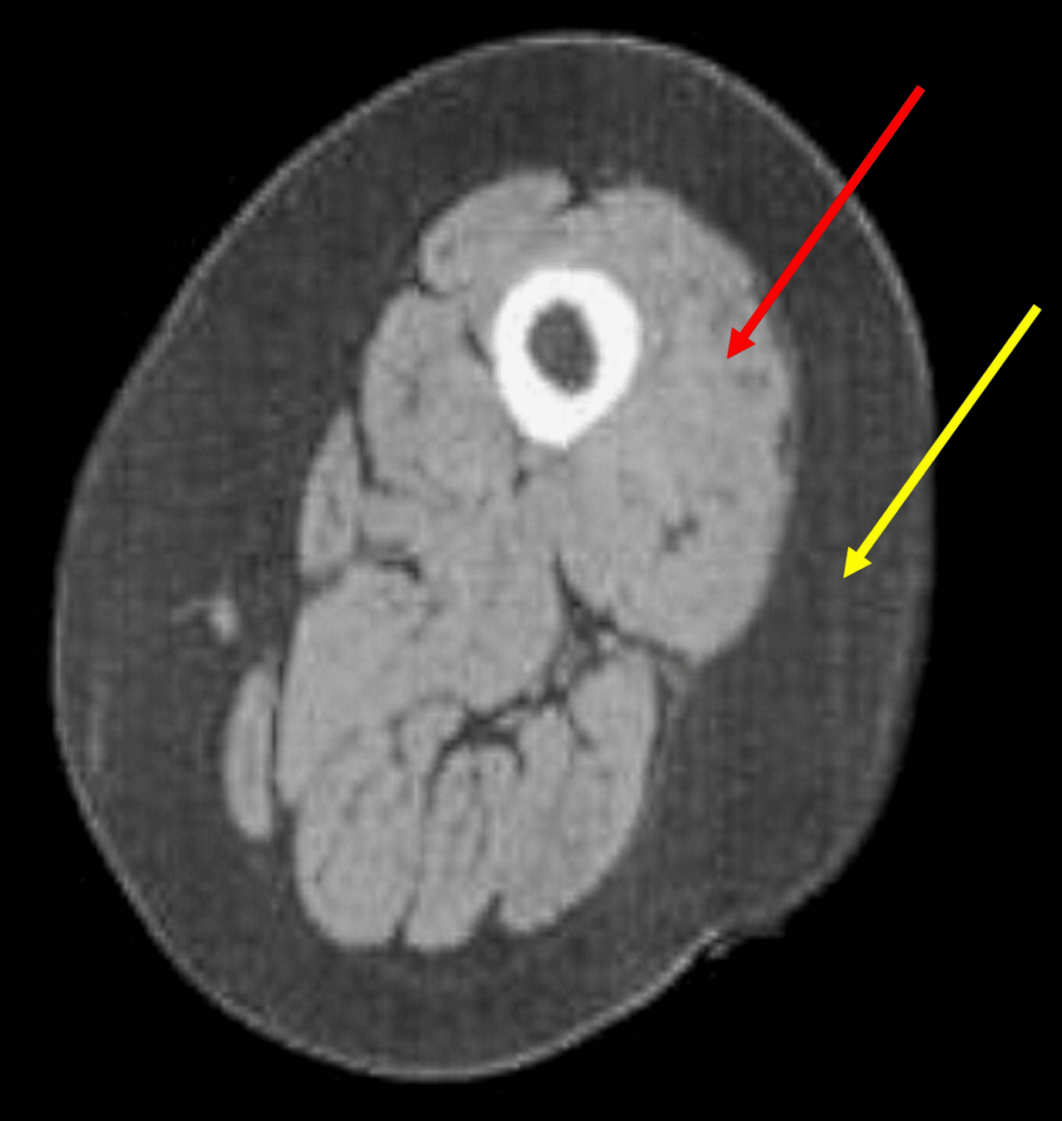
MRI shows sarcopenia of the thigh area of ​​a patient with COPD (with depressed FEV1, 50-79% of predicted). Hypotrophy (light gray area, red arrow) can be noted, with the presence of subcutaneous and intramuscular fat (dark gray area, yellow arrow) MRI: magnetic resonance imaging; COPD: chronic obstructive pulmonary disease; FEV1: forced expiratory volume in one second Image Credit: Bruno Bordoni
